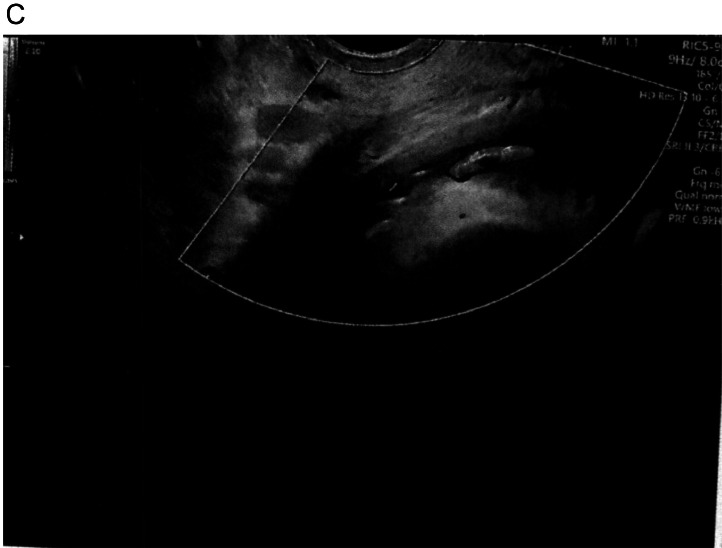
(A) Transvaginal grayscale ultrasound at 32 weeks demonstrating the cervix with vasa previa vessels visible as tubular anechoic structures traversing the membranes overlying the internal cervical os. (B) Transvaginal ultrasound demonstrating the velamentous vessels as linear hypoechoic structures coursing through the lower uterine segment membranes in close proximity to the internal os. The cervical canal is clearly visualized and (C) Additional transvaginal ultrasound view showing the relationship between the vasa previa vessels and the cervix. The unprotected fetal vessels are identified crossing the region of the internal cervical os, confirming the diagnosis prior to color Doppler confirmation.
